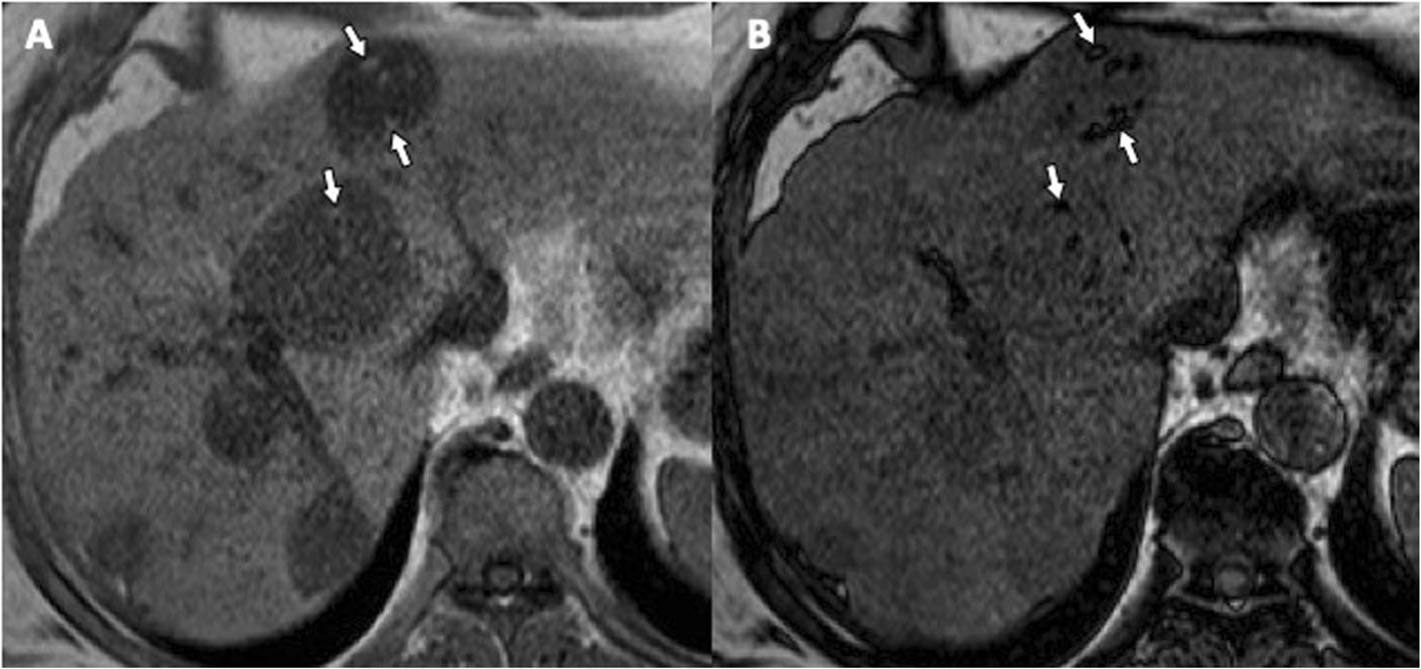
A 66-year-old man with multiple fat-containing hydatid cysts in the liver. a Axial gradient-echo in-phase T1-weighted MR image shows multiple high signal intensity foci (arrows) within hepatic hydatid cyst. b Axial gradient-echo opposed-phase T1-weighted MR image shows macroscopic fat within the same lesions, a finding confirmed by chemical shift artifact and signal cancelation (arrows) surrounding the lipid pure component
